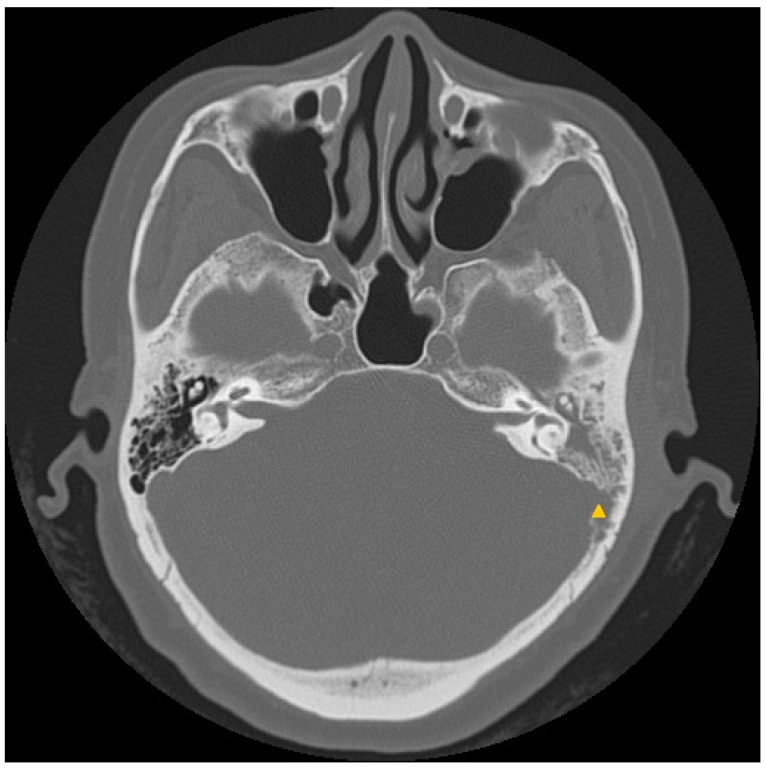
Case 1: A high-resolution computed tomography scan of the temporal bone shows erosion of the sigmoid plate (▲) with opacification of the left mastoid antrum, mastoid air cells, and middle ear cavity.
